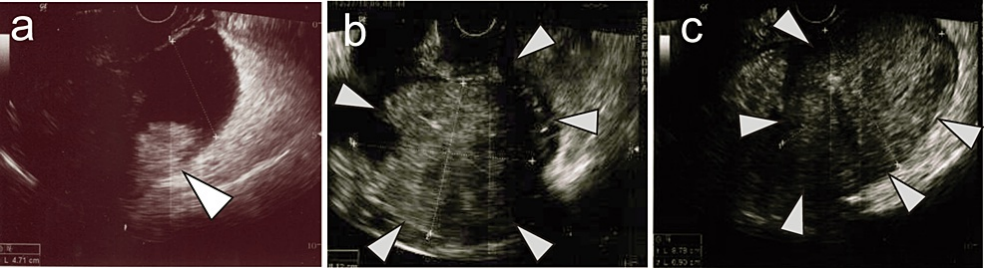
Transvaginal ultrasound findings after transvaginal oocyte retrieval (TVOR). (A) Hematoma was suspected in the inclusion cyst by ultrasound six days after TVOR (arrowhead). (B) Swollen ovary (arrowhead) was observed eight days after TVOR. (C) An inclusion cyst filled with hematoma was detected eight days after TVOR (arrowhead).
